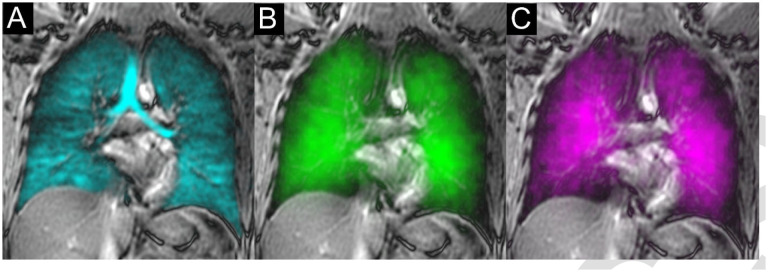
^129^Xe gas-transfer MRI in a 30-year-old male control
